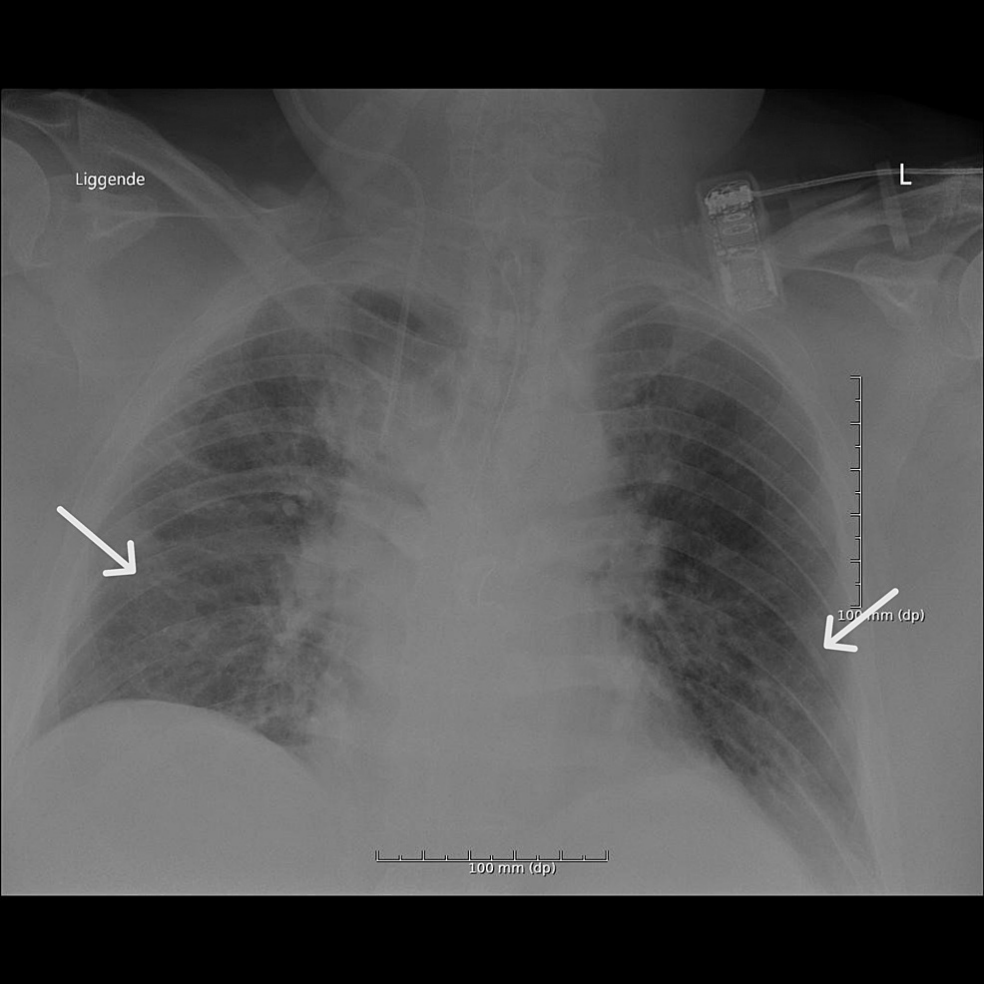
Chest X-ray on the day of admission showed bilateral patchy opacities.
